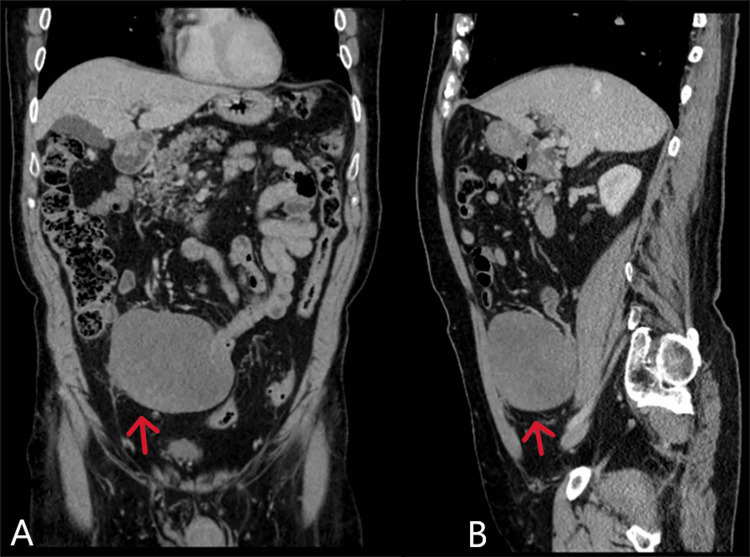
CT abdomen and pelvis (coronal section labelled A and sagittal section labelled B) demonstrating the mass in the right iliac fossa (red arrows).
